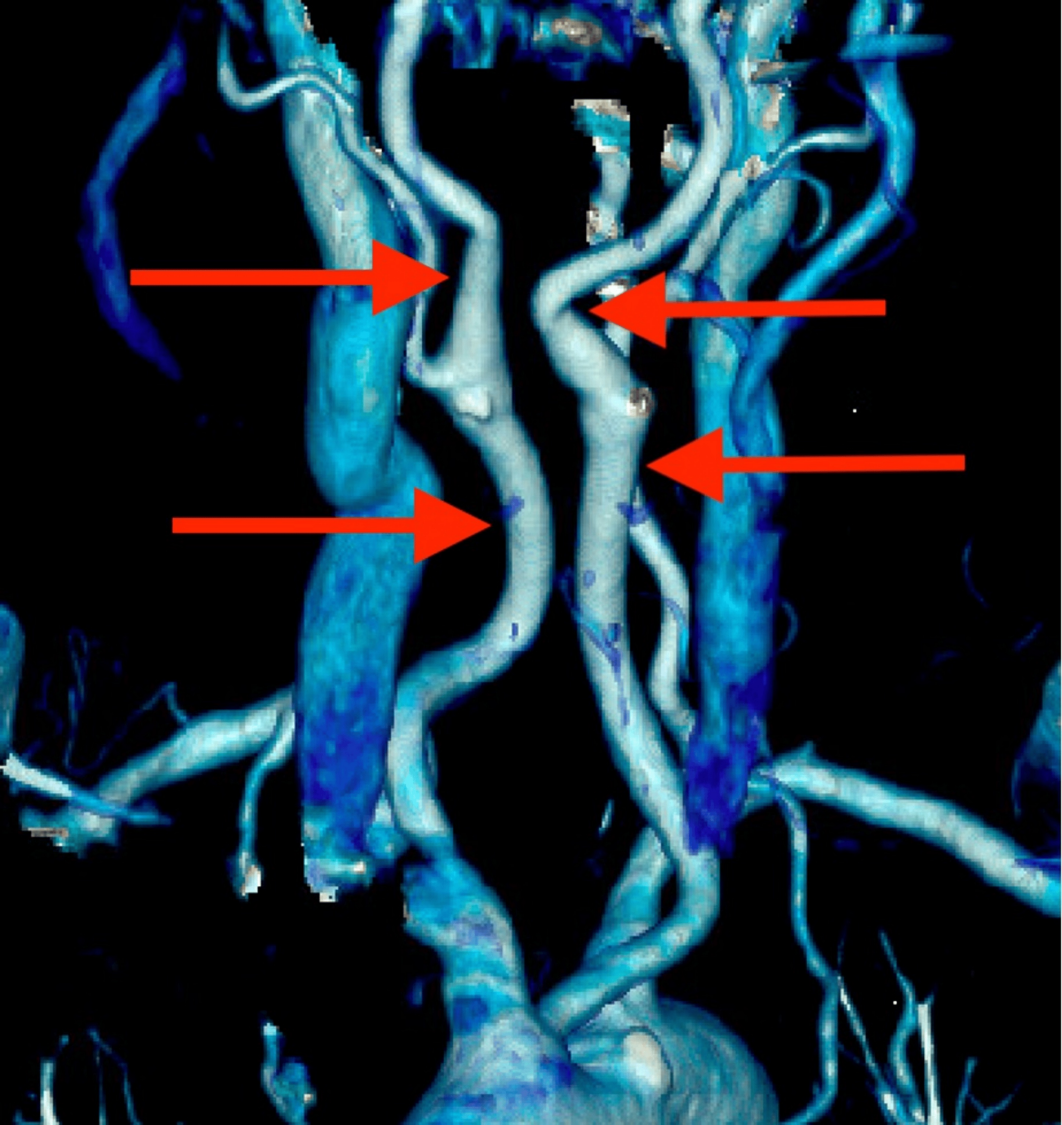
CT angiogram of the neck in coronal view demonstrating medialization of common and internal carotid arteries, indicated by the red arrows.
